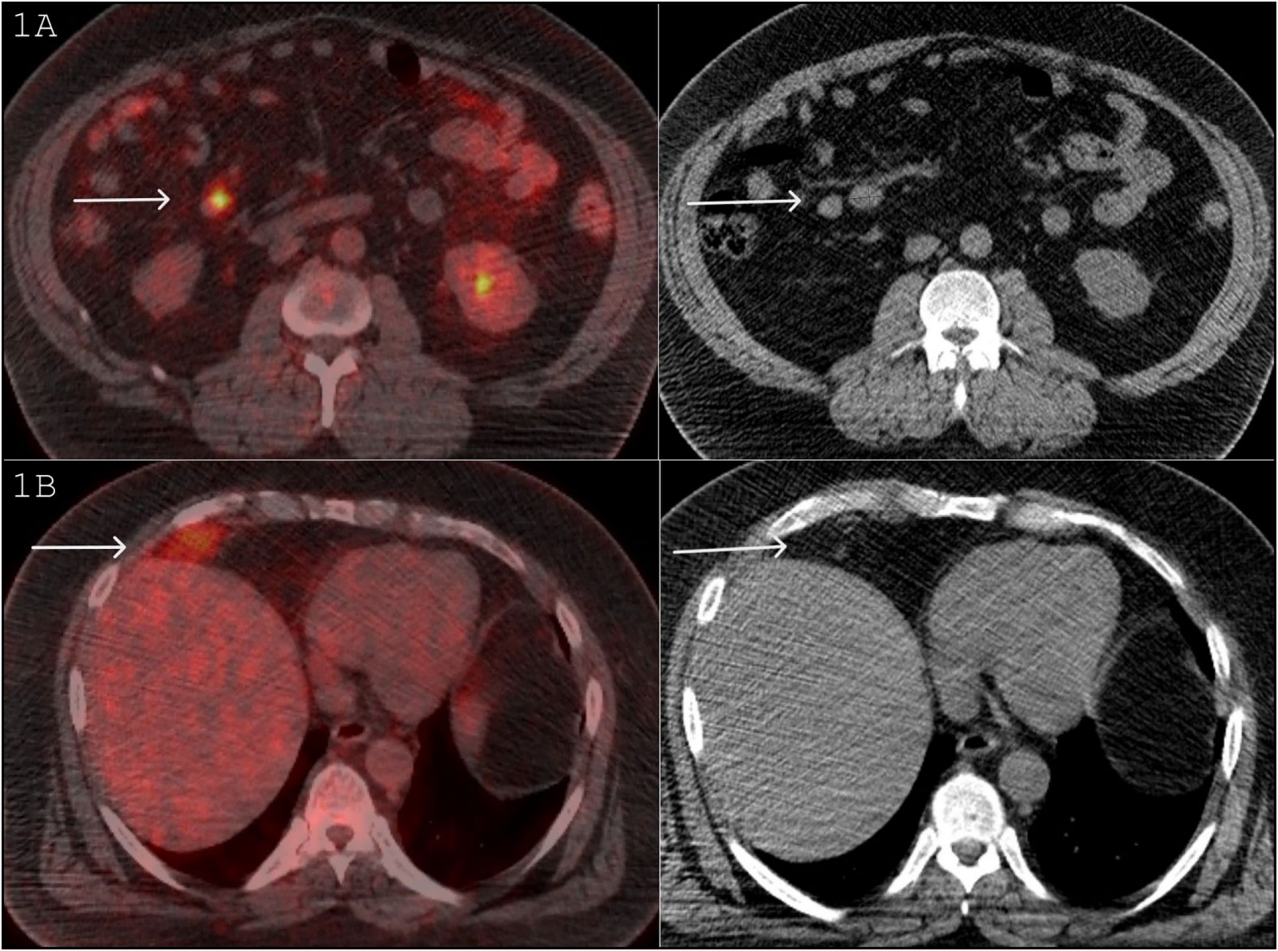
(A) FDG PET/CT November 2015: A 2.1 × 1.6 cm FDG avid lesion on the right side of the mesentery (SUV max 9.5, background hepatic SUV max 3.5), raising concerns for an abdominal melanoma metastasis. (B) FDG PET/CT November 2015: FDG avid mass in the right perihepatic location (SUV max 6.5). On the CT component, this mass is fat density and contains a large vessel.
